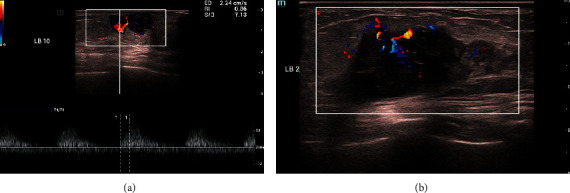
A-B 84-year-old woman with TNBC (invasive breast cancer). An irregular mass with indistinct margin and calcification. Color doppler ultrasound showed mixed and disorderly blood flow. (RI = 0.86).
